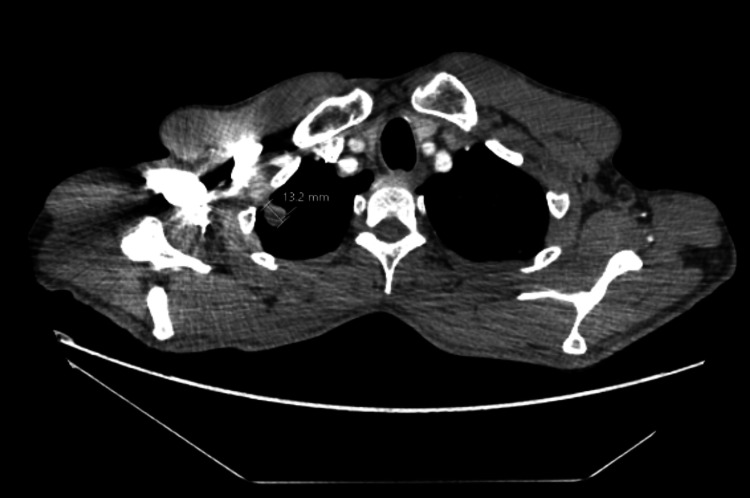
CT thorax with contrast (done six minths prior to current admission) showing a pulmonary nodule in the right upper lobe measuring in cross section 13.2 mm
